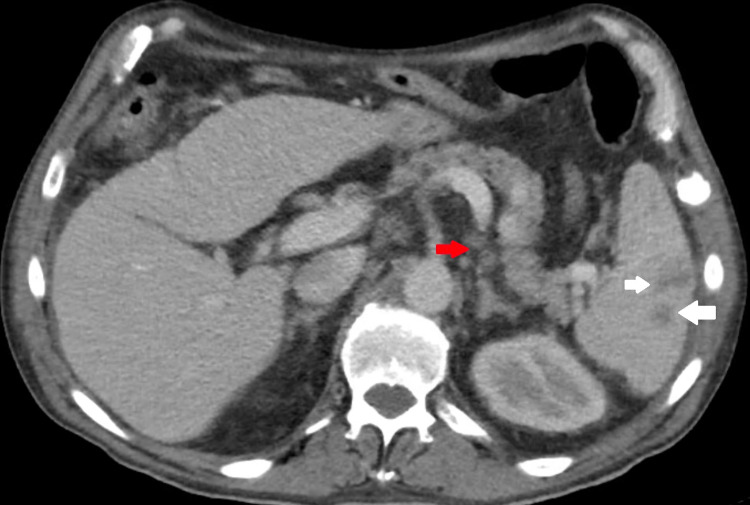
CT abdomen showing the presence of mesenteric and retroperitoneal adenopathy (red arrow) and multiple ill-defined splenic lesions (white arrows) CT: computed tomography
